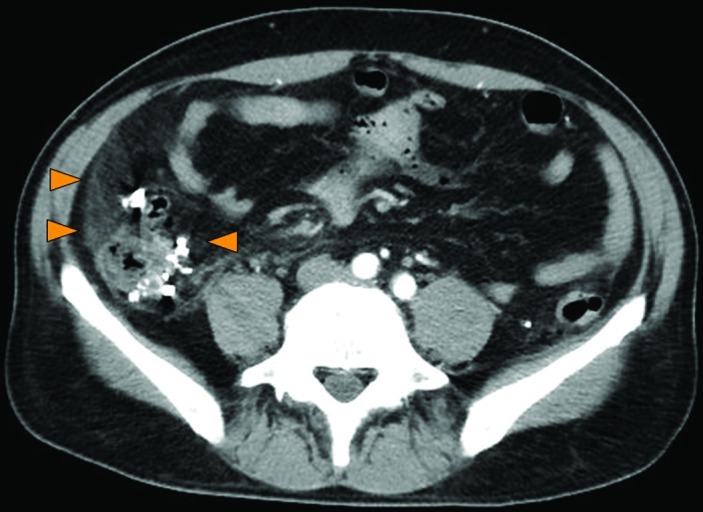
Abdominal computed tomography (CT) findings on admission. CT imaging revealed thickening of the ascending colon wall, numerous diverticula within and outside the colon wall and thickening of the surrounding fat layer (arrowheads).
